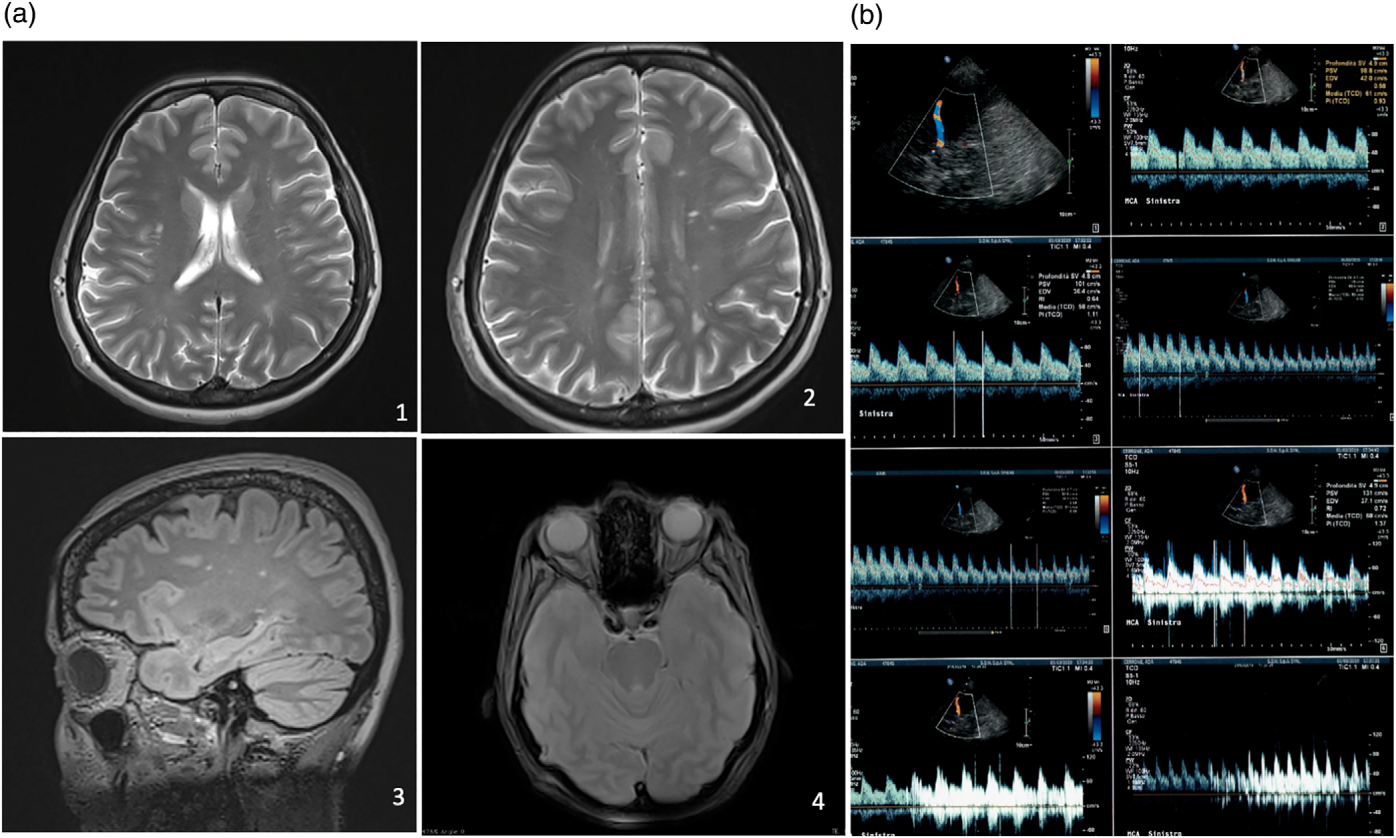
(a) Magnetic resonance imaging (MRI) of the brain and brainstem with and without intravenous paramagnetic contrast showed: (1)–(2) T2W TSE FSE TRA gliotic areas of the white substance of the brain; (3) 3D FLAIR SAG SAT gliotic areas in of the white substance of the brain. (4) GRADIENT AXIAL asymmetry and tortuosity of carotid siphons. (b) Transcranial Doppler Ultrasonography (TCD) with the agitated saline test showed a high degree of shunt with uncountable microembolic signals (the “curtain effect”)
